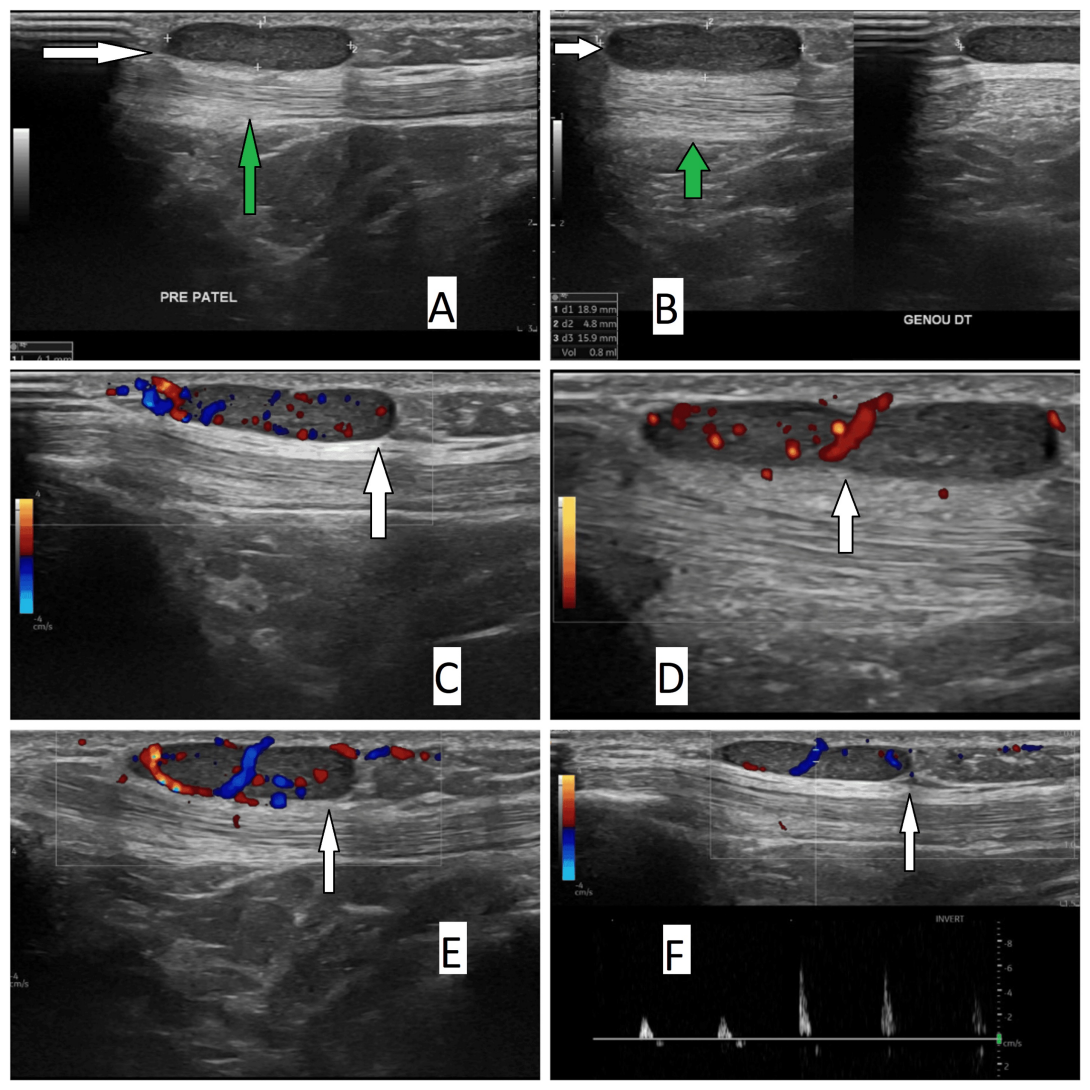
Preoperative scans of the second patient Preoperative echography (A, B) and echo-Doppler (C, D, E, F) images (white arrow: the mass, green arrow: the patellar tendon).
